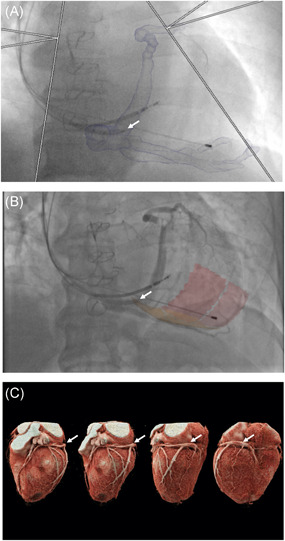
CT‐derived coronary venous anatomy. (A) Overlaid onto fluoroscopy to aid operator CS cannulation. Guide catheter (arrowed) entering CS ostium. (B) Balloon venogram of corresponding coronary venous anatomy with target segments overlaid onto fluoroscopy. (C) Volume‐rendered cardiac CT angiography series delineating coronary venous anatomy (arrowed). CS, coronary sinus; CT, computed tomography
